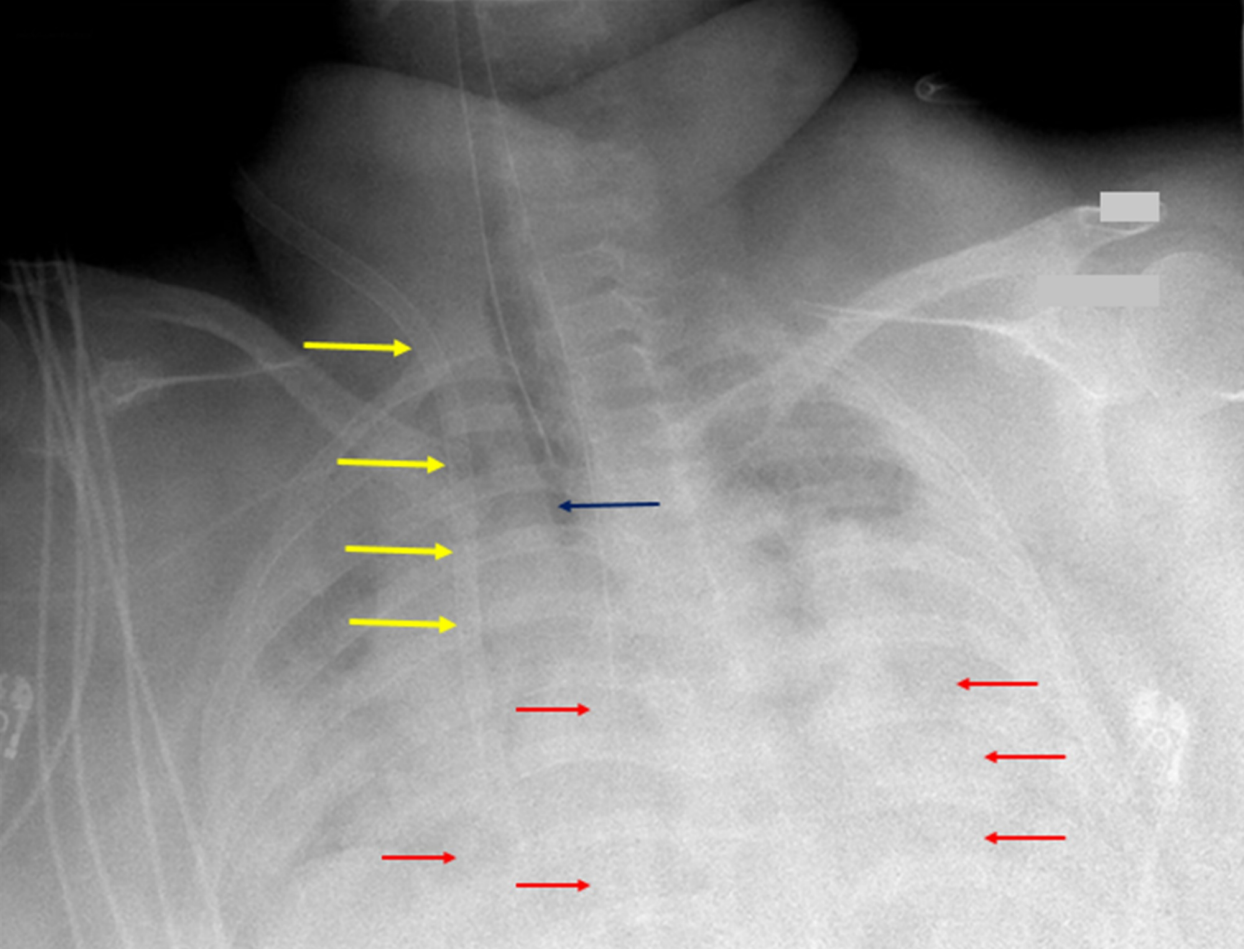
Final chest radiograph A final chest radiograph shows that pneumothoraces are not present. The imaging study continues to reveal persistent bilateral opacities (red arrows) as before, consistent with acute respiratory distress syndrome. A 20 French extracorporeal membrane oxygenation catheter is shown (yellow arrows) over the right lung field, terminating in the right atrium. The patient’s airway is patent, and is demonstrated by the dark blue arrow.
